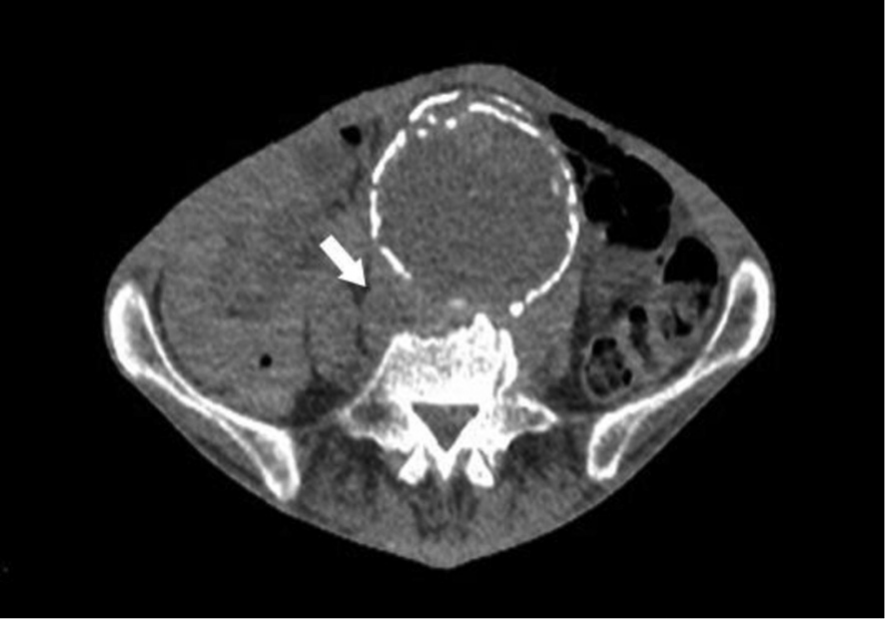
Non-contrast axial CT image showing an aneurysm with erosion of the anterior vertebral body and a soft tissue collection posterior to the aneurysm between the psoas muscle and the spine (arrow).
